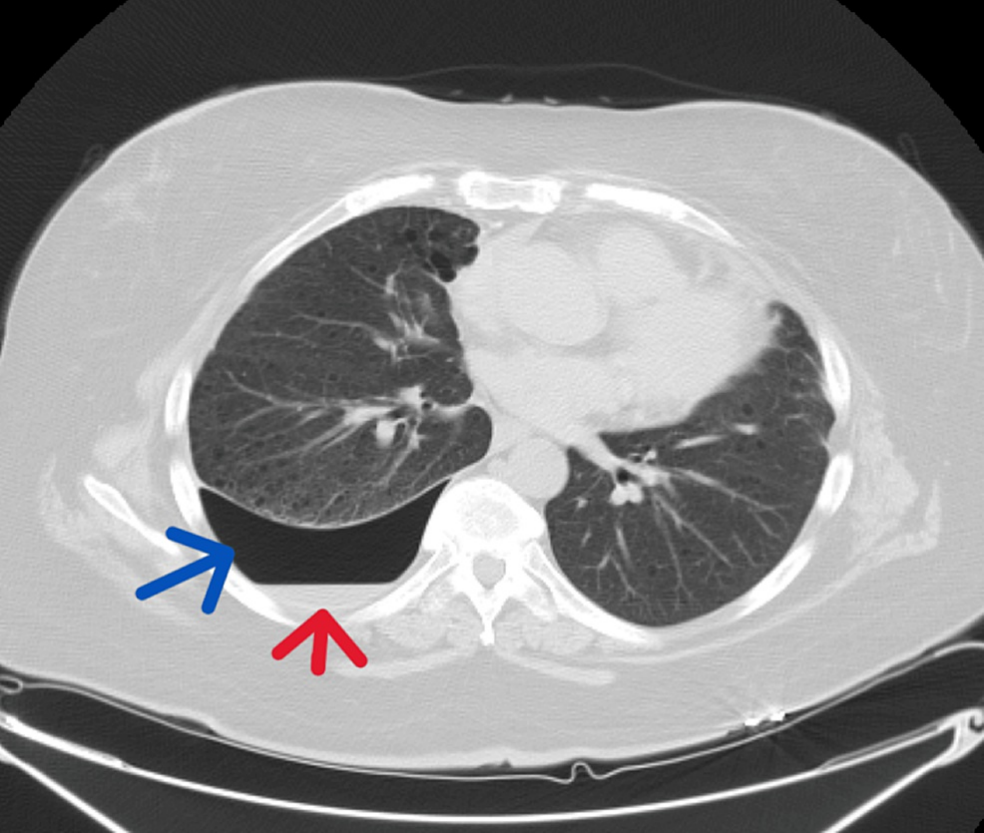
CT of the chest showing right-sided hydropneumothorax (the red arrow shows hydrothorax and the blue arrow shows pneumothorax). It also shows cystic lungs consistent with lymphangioleiomyomatosis.
